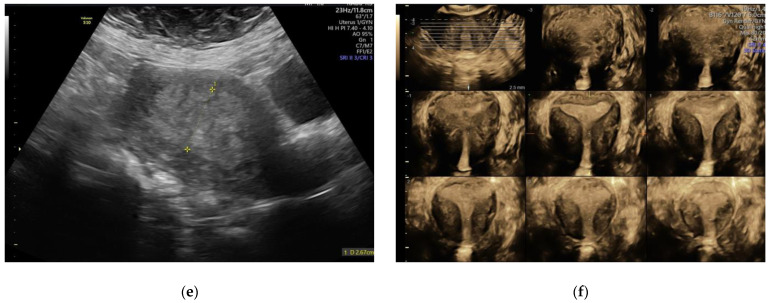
Ultrasonographic images. (a) EH—the endometrial line is not clearly defined, discreet hyperechogenic aspect of the endometrium (endometrial thickness = 25.45 mm); (b) EH—2D Color Doppler. Dispersed endometrial vessels and irregular endo-myometrial junction can be observed; (c) 2D Color Doppler—dispersed endometrial vessels, hyperechogenic endometrium without an endometrial line can be seen; (d) echogenic aspect of the endometrial fluid. A lesion can be observed (polyp), with an implant base of less than 25% of the endometrial surface; (e) endometrial adenocarcinoma. Irregular and infiltrative aspect of the endometrium especially in the endo-myometrial junction, endometrial thickness of 26.7 mm; (f) 3D TUI (Tomographic Ultrasound Imaging) Image acquisition.
